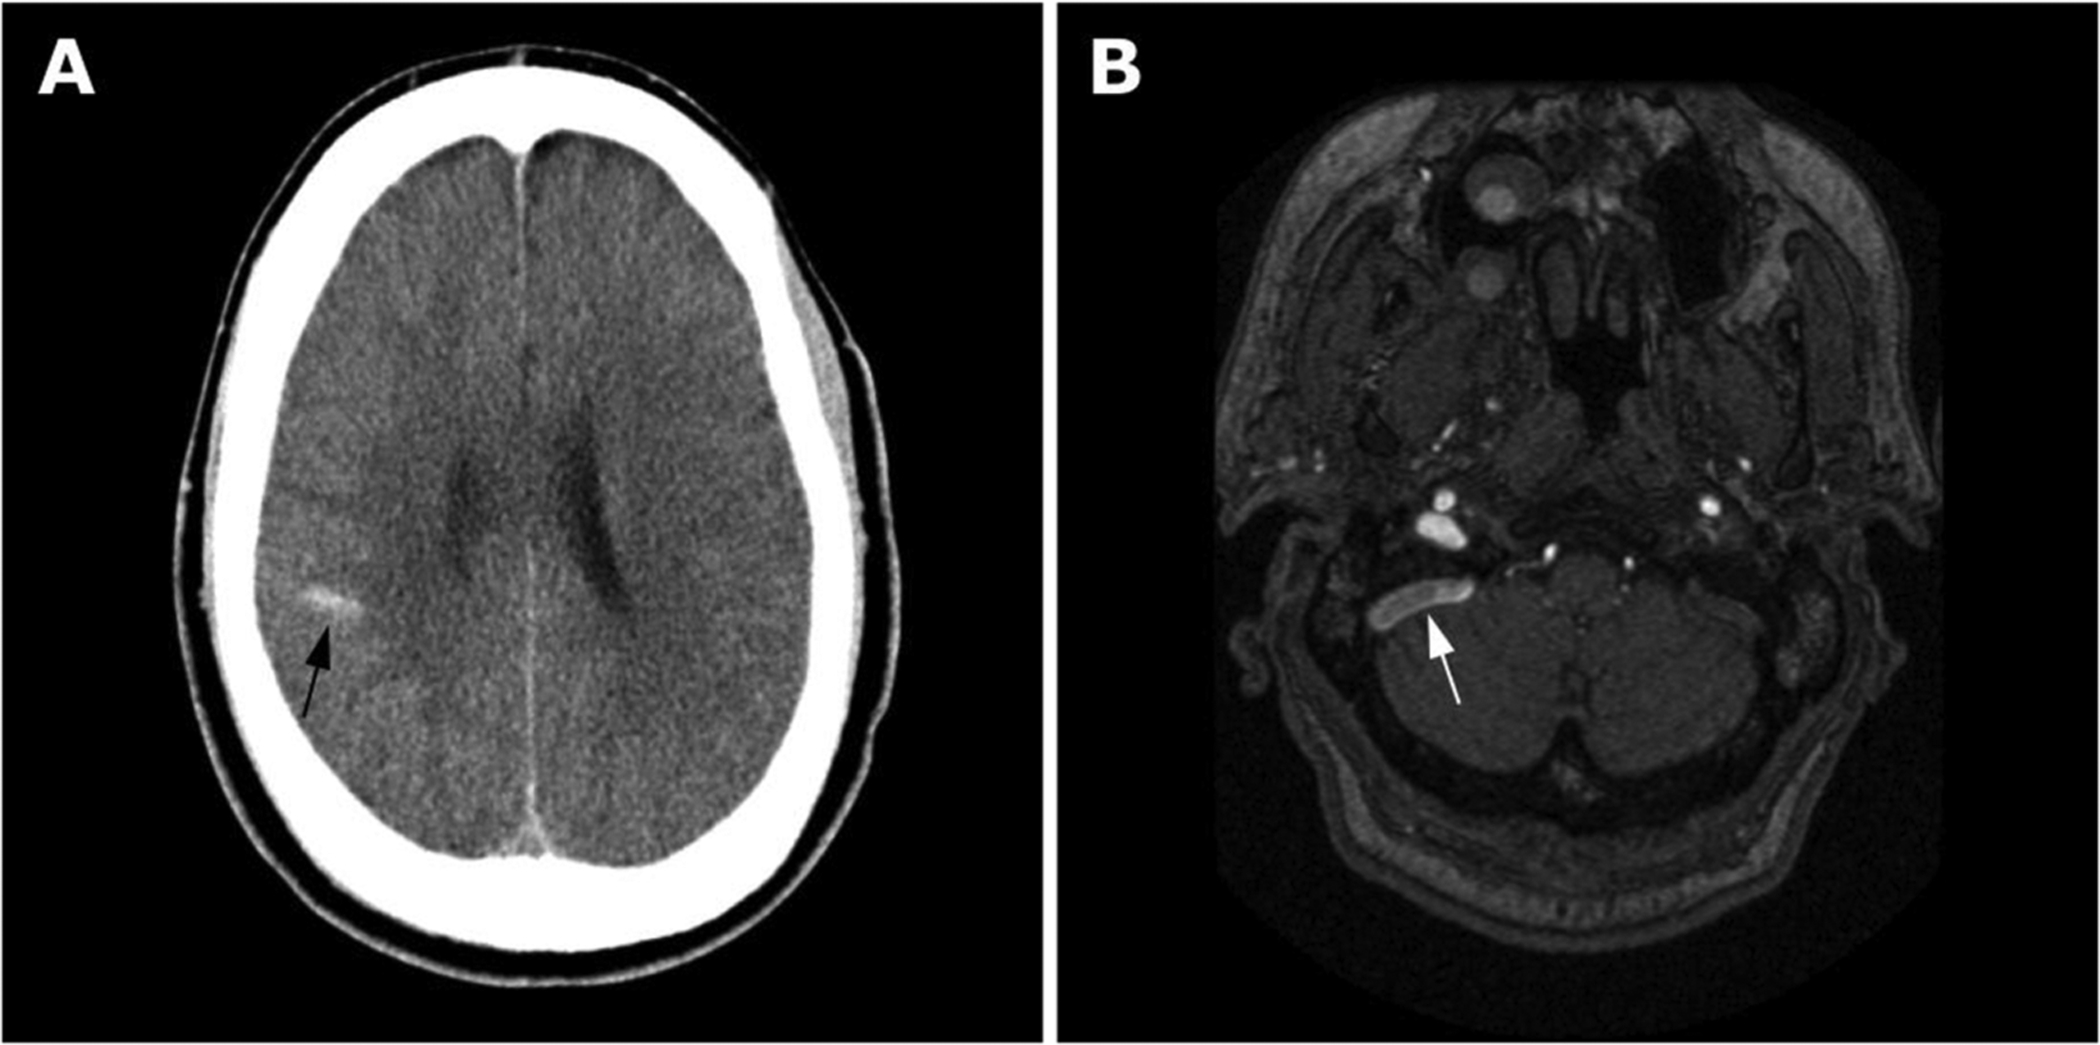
(A) Axial head CT reveals right posterior temporal-parietal hemorrhage (black arrow). (B) Axial time-of-flight MRA demonstrates flow-related enhancement in the right sigmoid sinus (white arrow) consistent with venous reflux in cranial direction.
